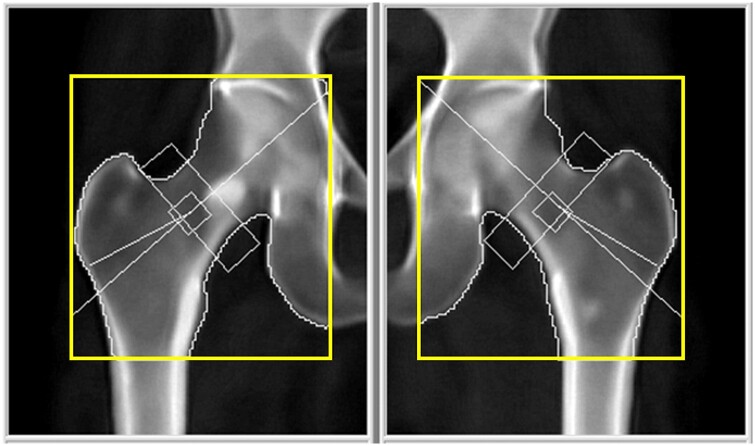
DXA scan of 6-month follow up that re-processed and analysed correctly, the T-scores of the right and left femoral neck were recorded as -1.5 and -2.0, respectively; while d_0_ of the right hip was 54.4, left hip was 53.4. DXA = dual-energy X-ray absorptiometry.
